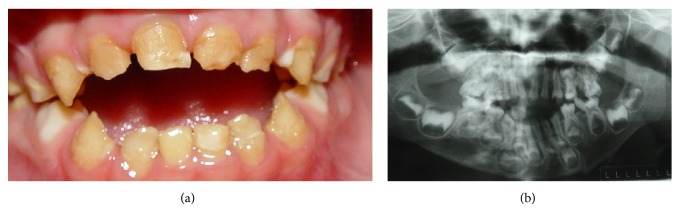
(a) Frontal view of amelogenesis imperfecta; (b) panoramic radiography before treatment.
